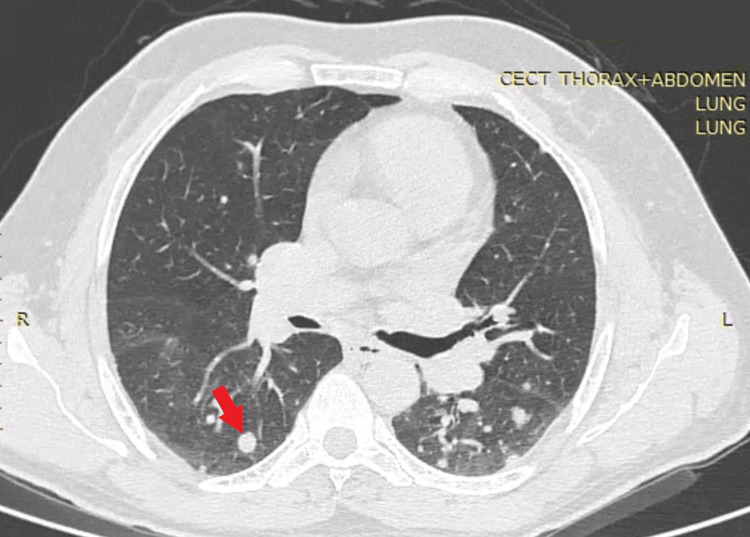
CT Chest before starting chemotherapy showed bilateral parenchymal metastatic nodules(red arrow)
